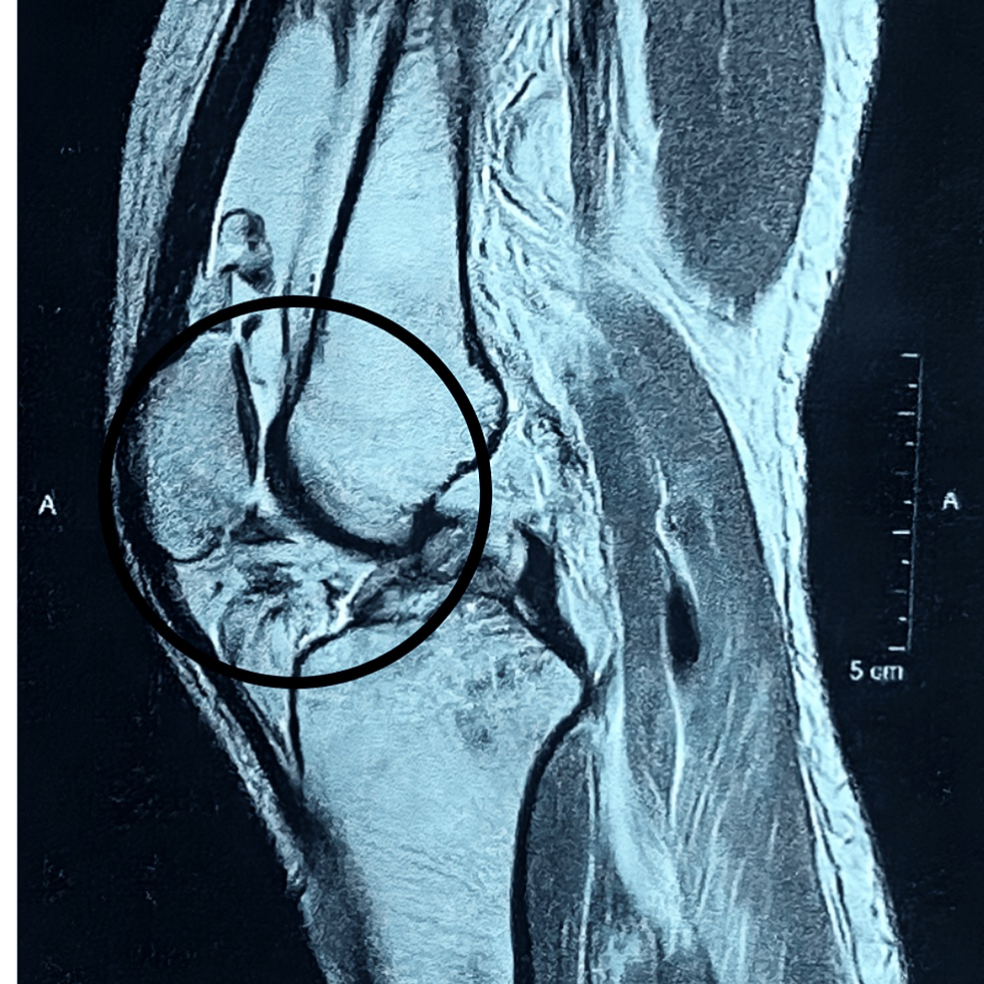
MRI showing ACL tear MRI: Magnetic resonance imaging; ACL: Anterior cruciate ligament
